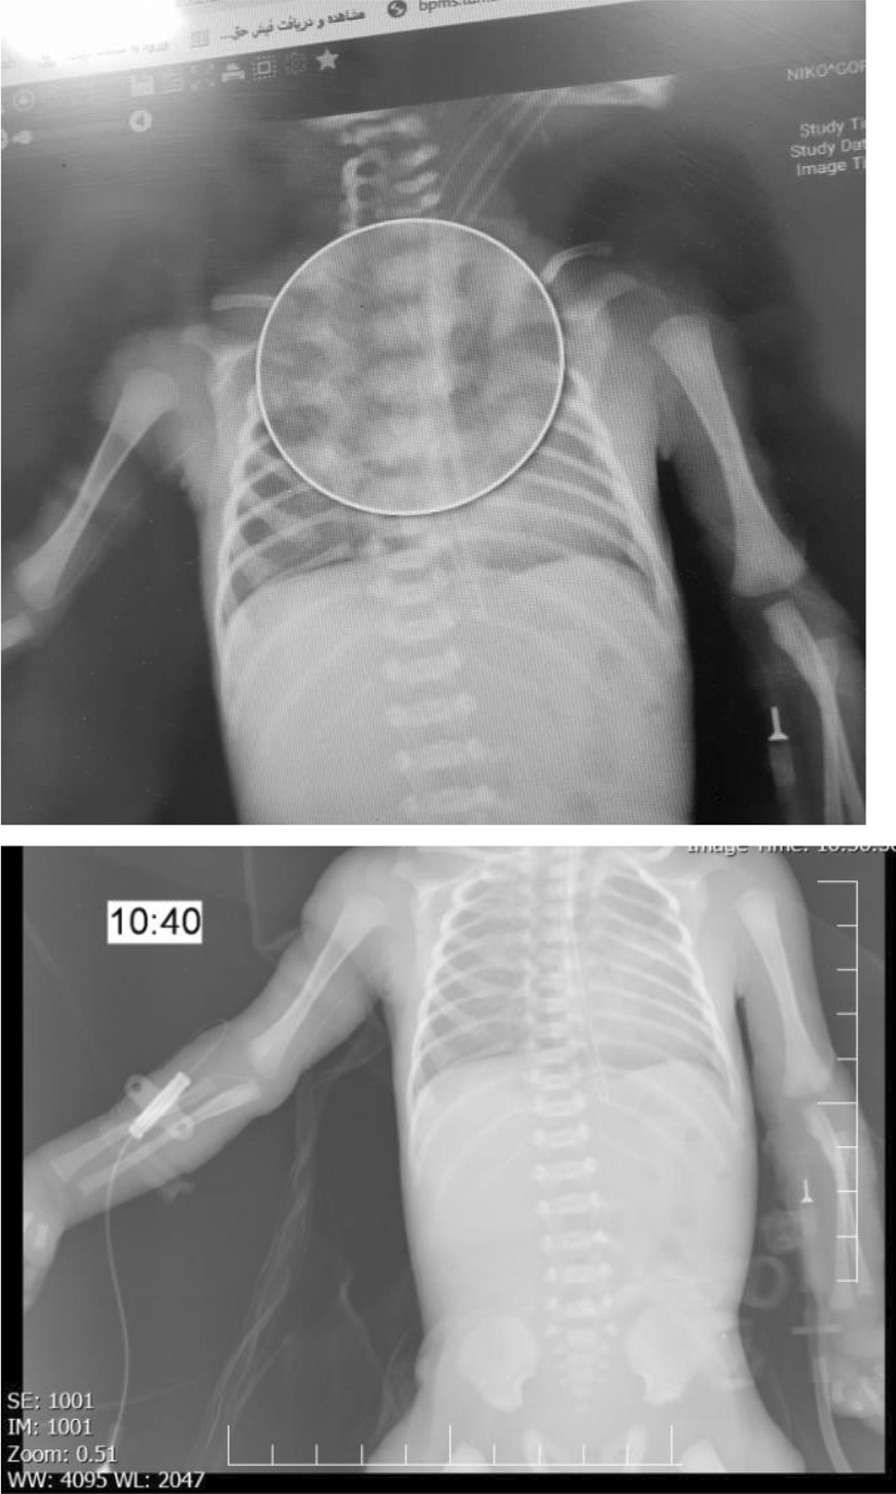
Chest X-ray findings showing the tip of the peripherally inserted central catheter line at appropriate position
